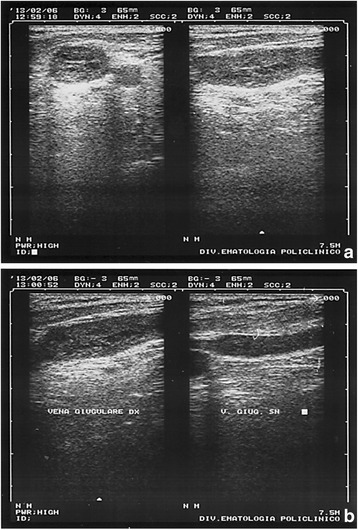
Jugular vein echography. a Right internal jugular vein thrombosis, in transverse and longitudinal sections. A heterogeneous echogenic material fills and distends the right internal jugular vein; b comparison of the right and left internal jugular veins. Ultrasonography shows different diameter between the two vessels
